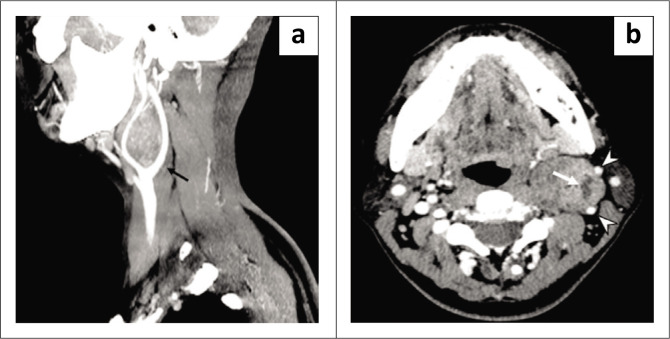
(a) Contrast-enhanced CT neck sagittal view in maximal intensity projection (MIP) showing a well-defined, heterogeneously enhancing mass at the left carotid bifurcation resulting in splaying of the left internal and external carotid arteries in keeping with lyre sign (black arrow). (b) Contrast-enhanced CT neck axial view. Note the internal cystic component (white arrow), lateral displacement of the left internal and external carotid arteries (white arrowheads) with non-visualisation of the left internal jugular vein likely due to compression by the mass.
